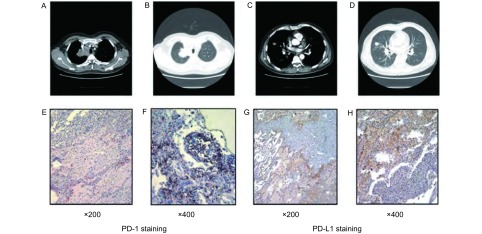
肺类癌患者的胸部CT和其肺癌组织中PD-1/PD-L1表达情况。A-B：右肺上叶见一大小为4 cm×5 cm肿物，侵及上腔静脉；C-D：右肺中叶亦见一大小为2 cm×2 cm肿物；E-F：PD-1表达呈强阳性(200×/400×)；G-H：PD-L1表达呈强阳性(200×/400×)。 The chest CT scan and the expression of PD-L1 and PD-1 for the patient who died due to pulmonary carcinoid. A-B: There was a tumor size of 4 cm×5 cm mass in the right upper lobe, which invaded the superior vena cava; C-D: There was also a tumor size of 2 cm×2 cm lesion in the right middle lobe; E-F: the strongly positive expression of PD-1 (3+; 200×/400×); G-H: the strongly positive expression of PD-L1 (3+; 200×/400×).
